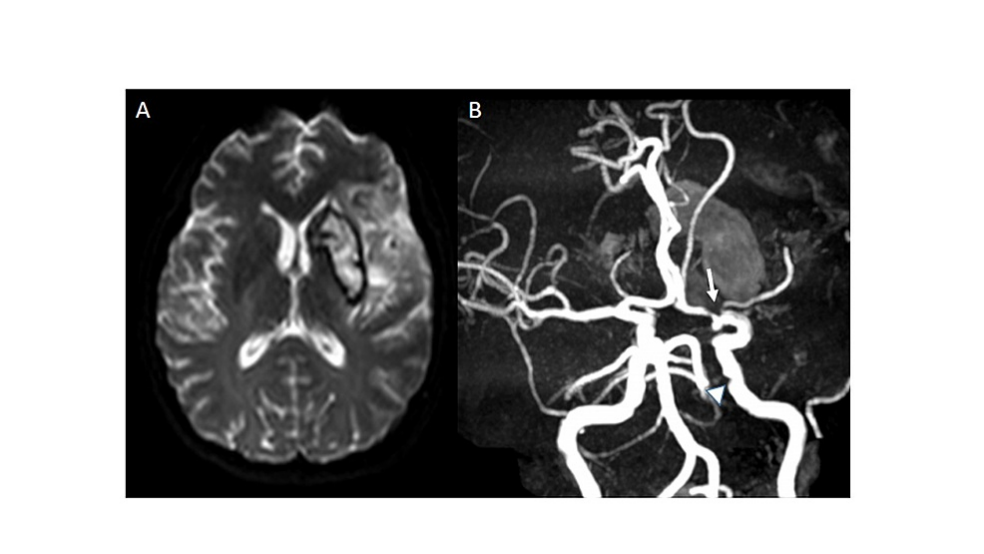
Acute ischemic stroke (A) Brain MRI, apparent diffusion coefficient map protocol shows subacute cerebral infarction in the territory of the left MCA. (B) MRA shows an occlusion in the M1 segment of the left MCA (arrow). In addition, there is evidence of alteration in the morphology of the intracranial wall of the ICA ipsilateral to the lesion (arrowhead). MRI, magnetic resonance imaging; MCA, middle cerebral artery; MRA, magnetic resonance angiography; ICA, internal carotid artery.
